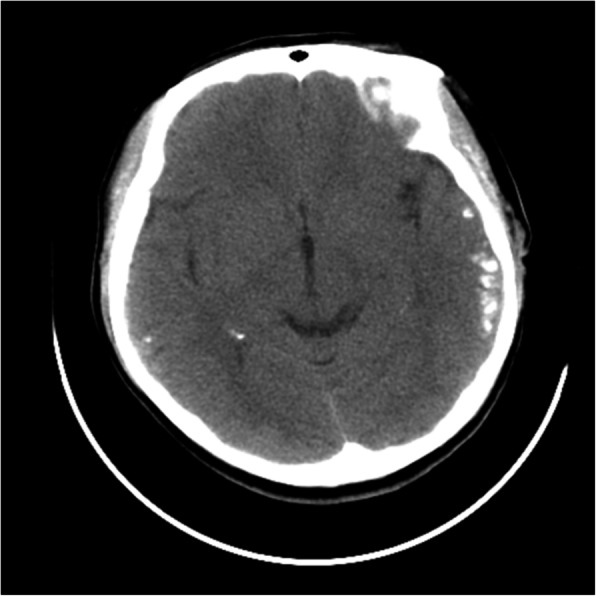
Plain computed tomography of the brain at presentation to the hospital showing patchy calcification in bilateral temporal cortico-subcortical areas (left > right)
